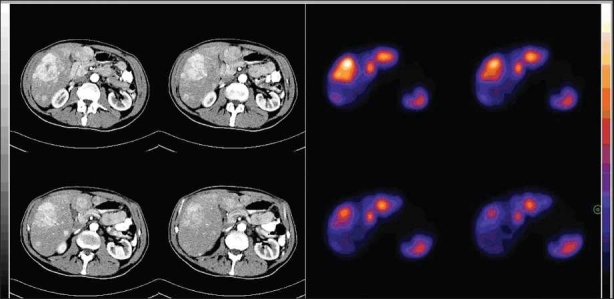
An image fusion in which positive uptake of activity in SPECT image (right) corresponds to NET lesions in the liver at CT image (left). There are also corresponding physiological uptakes in gall bladder and left kidney (left posterolateral aspect). Right kidney has no uptake in the SPECT study
